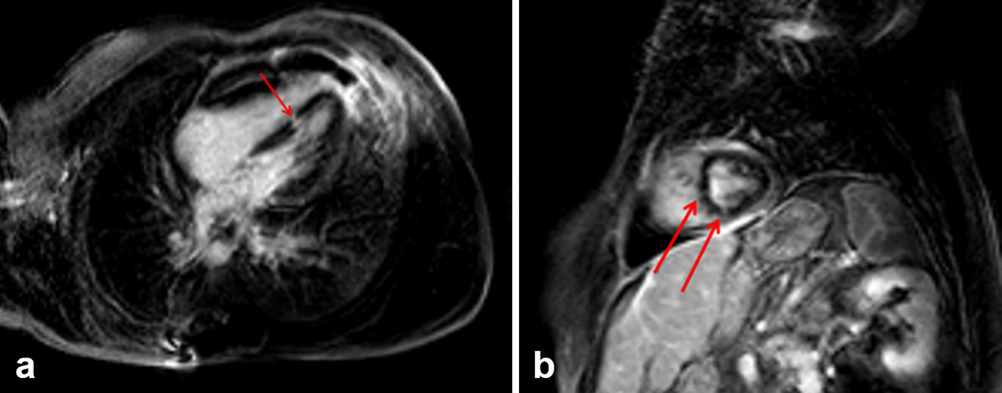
(a) MRI delayed-enhancement sequence (four-chamber view), showing patched enhancing area in the interventricular septum (arrow). (b) MRI delayed-enhancement sequence (short-axis view), showing patched enhancing area in the lower and anterior ventricle walls (arrows).
